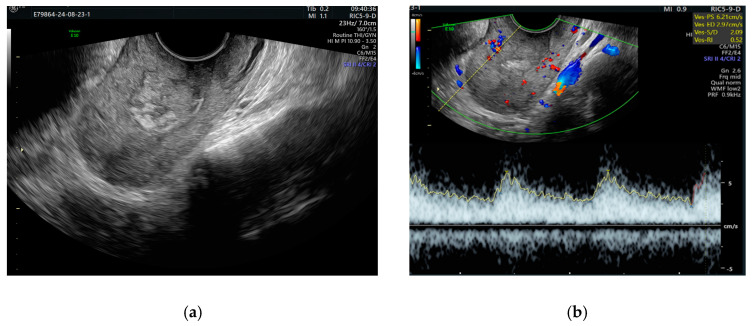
Vaginal ultrasound 4 weeks after embolization shows (a) intrauterine heterogeneous contents; (b) normal vascular indices.
